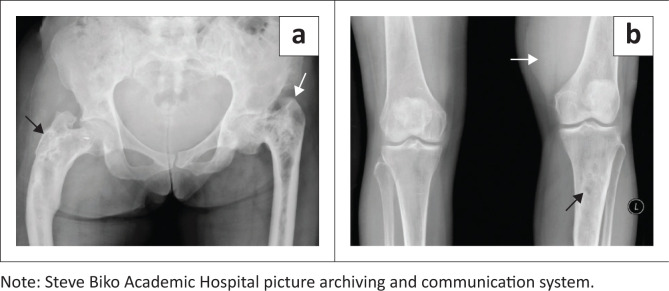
Anterior-posterior (AP) radiograph of the pelvis (a) demonstrates mixed lytic, sclerotic, expansile lesions (white arrow) with associated varus angulation and bowing, and shepherd crook deformity of the proximal femur (black arrow). AP radiograph of the knees (b) shows a similar lesion within the left proximal tibia (black arrow) with a soft tissue mass adjacent to the left medial distal femur (white arrow).
